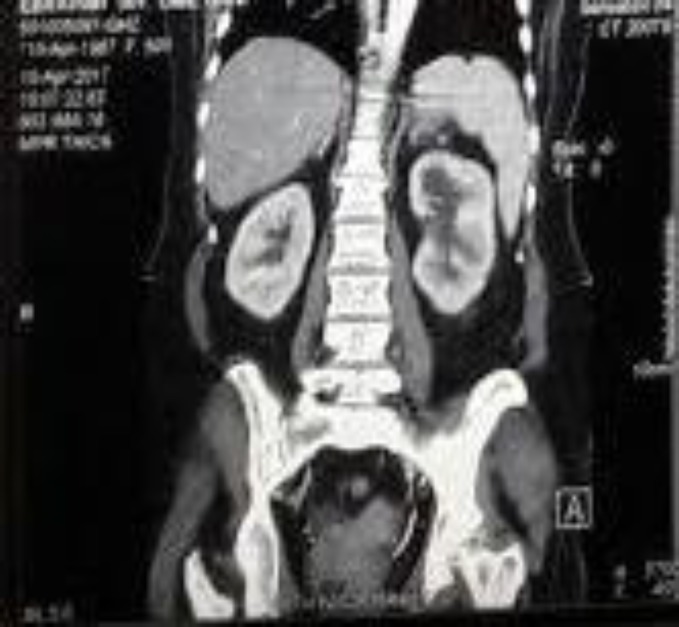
Abdominal and pelvic computed tomography, gas bubble in both renal parenchyma and surrounding fat stranding
